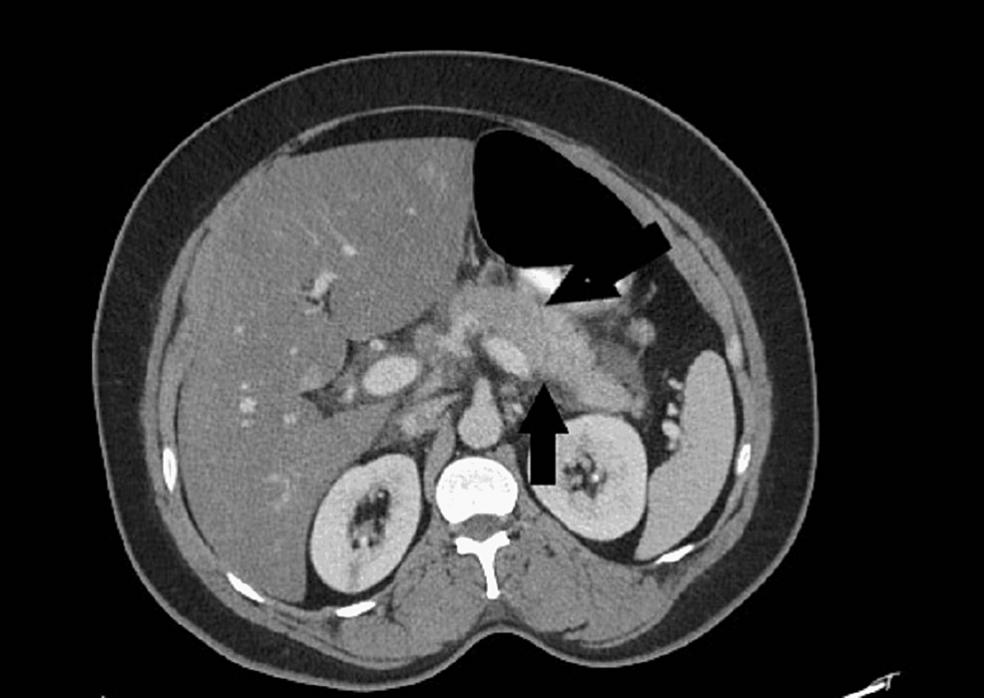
CT scan abdomen showing irregular pancreatic contour and diffuse peri-pancreatic swelling.
